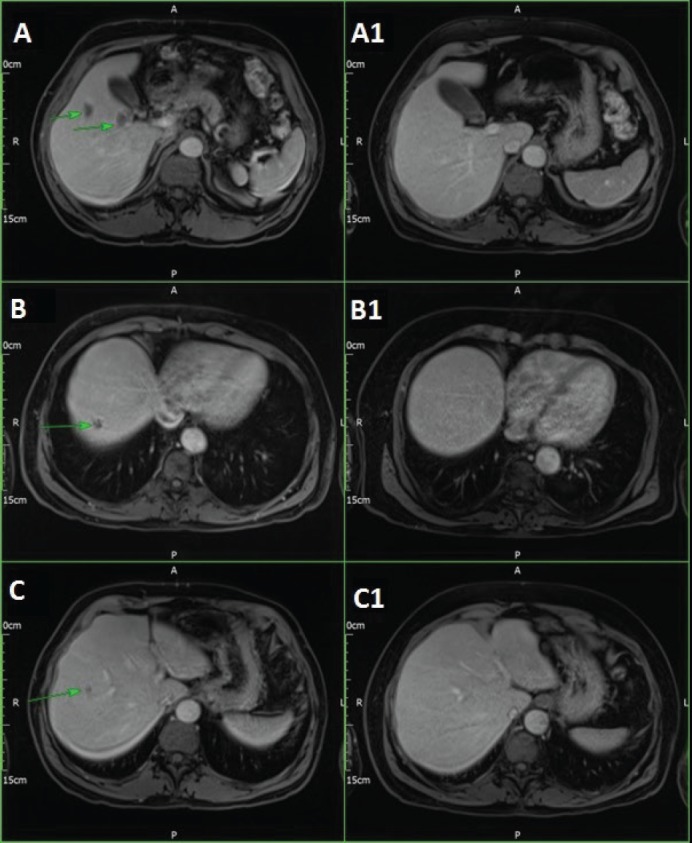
Panitumumab-induced fatal ILD. MRI liver, March 2016 (A, B, C) and September 2016 (A1, BA, C1).
